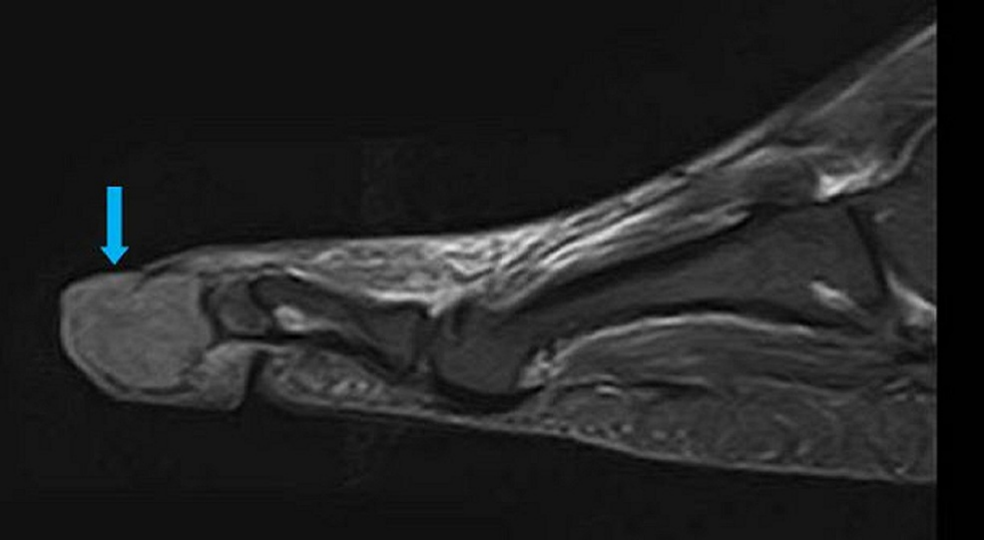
Sagittal view on the MRI T2 STIR sequence visualizing the mass on the distal phalange of the fourth toe (blue arrow) STIR, short tau inversion recovery
